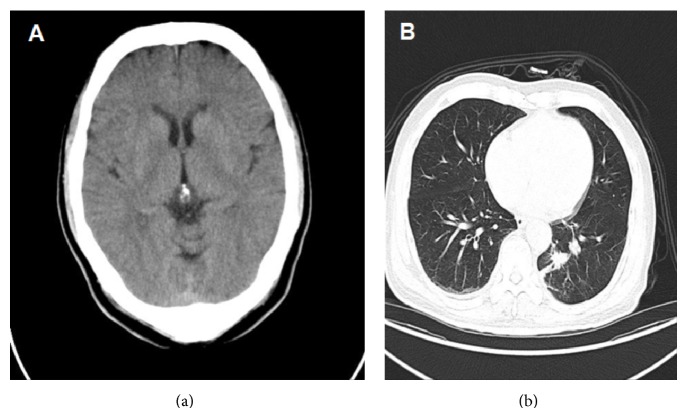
(a) Cranial CT produced no unusual findings. (b) Enhanced chest CT showed a mass with irregular border in the left to middle mediastinum.
